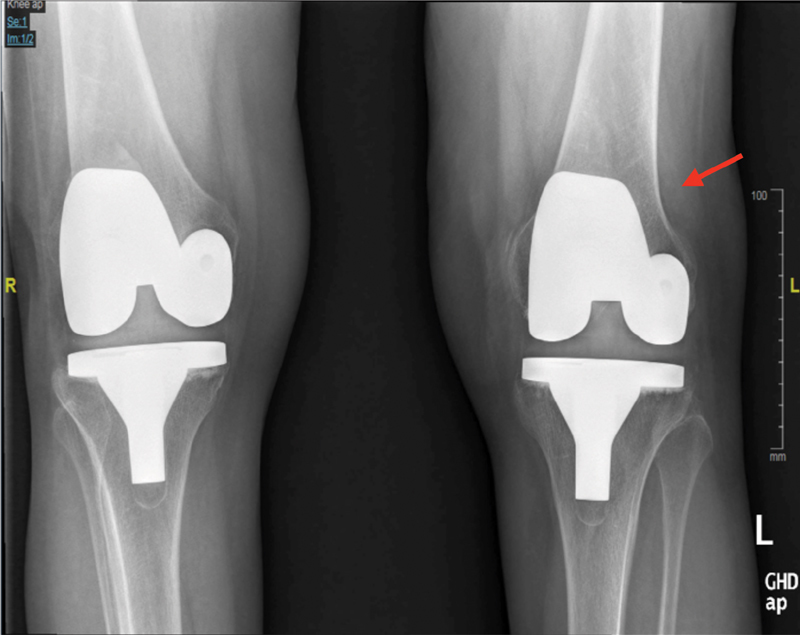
Postoperative X-ray of both knees, showing right femoral implant was placed in the left knee (arrow).
